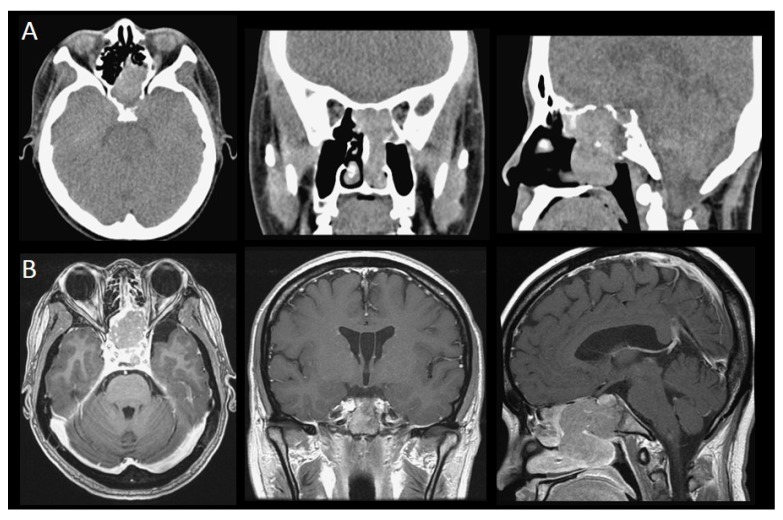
CT and MRI images of sinonasal metastatic cancers: (A) A 37-year-old female diagnosed with metastatic retroperitoneum leiomyosarcoma. The CT scan revealed that the tumor involved the nasal chamber, bilateral sphenoid sinus, left side of the posterior ethmoid sinus, left aspect of the sellar floor, and the clivus. (B) A 45-year-old female diagnosed with retrorectal neuroendocrine carcinoma. The MRI scans revealed that the tumor involved the sphenoid sinus, sella, suprasella, left cavernous sinus, and pituitary gland.
